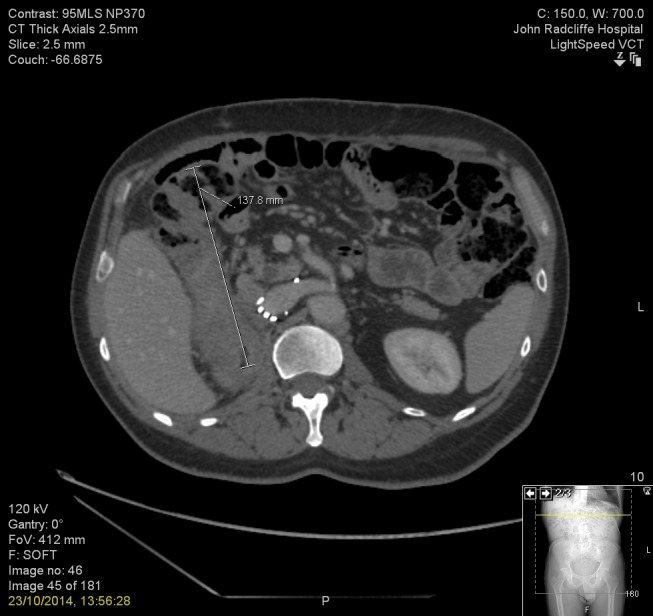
CT images showing mass centered on ascending colon and hepatic flexure.
